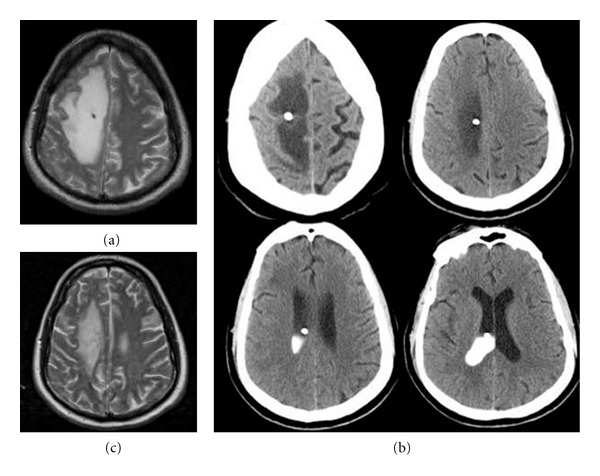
Patient A's imaging: (a) T2 MRI of the brain on admission. (b) Preoperative ventriculogram, performed through the ventricular reservoir. There is contrast within the ventricle, but it does not pass into the catheter track or the edematous white matter surrounding the catheter. (c) T2 MRI on postoperative day eight demonstrating decreased area of hyperintensity in the white matter compared to preoperative imaging.
